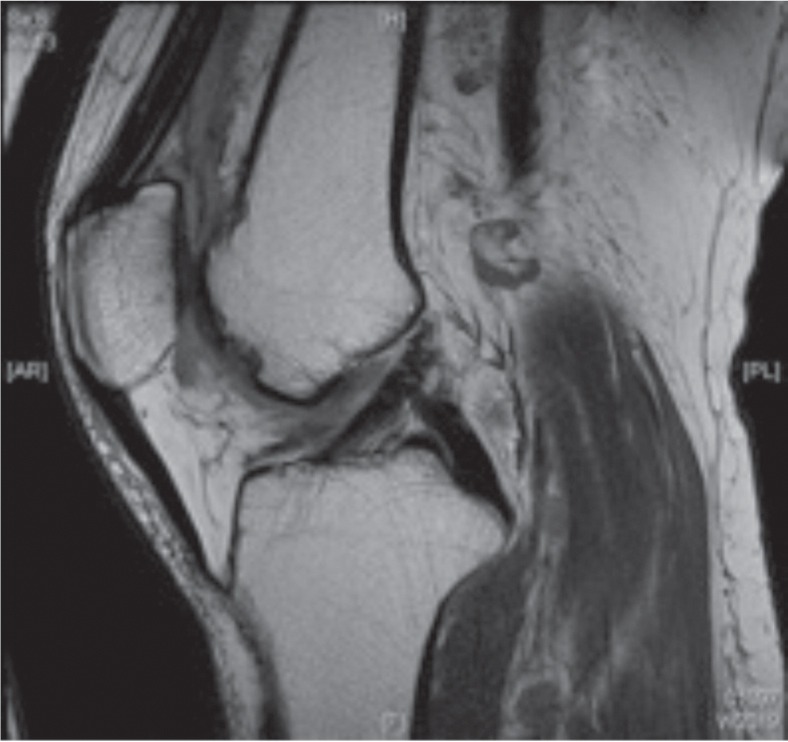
Osteochondral defect over femoral sulcus on MRI.
